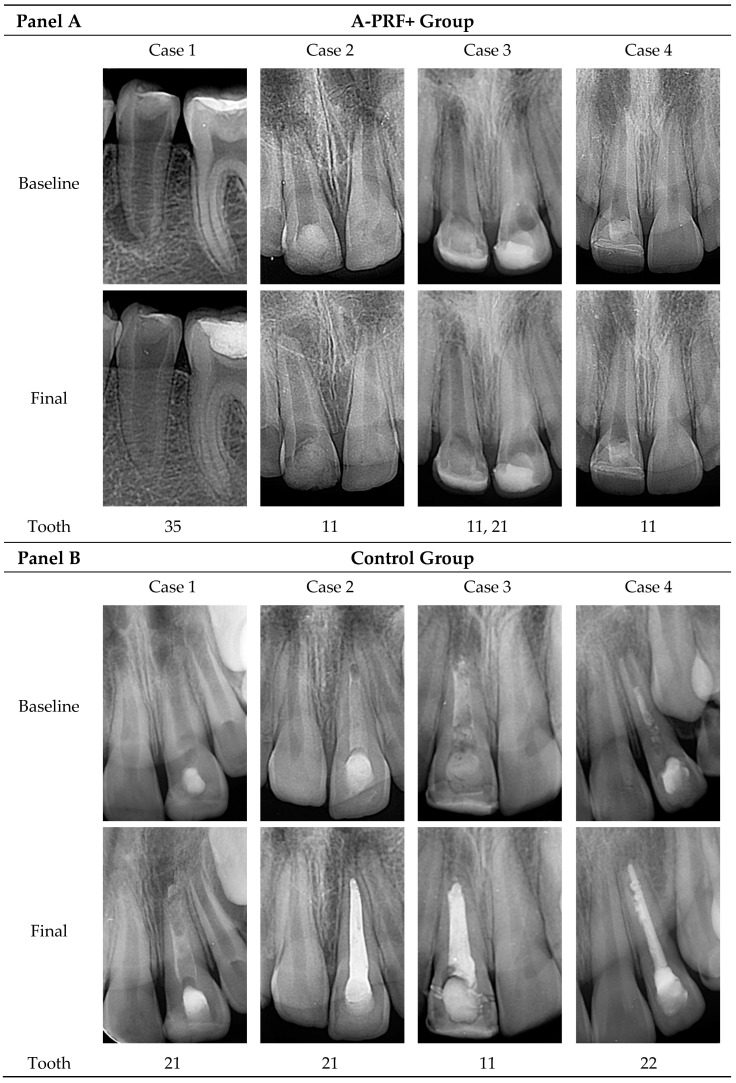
Representative periapical radiographs of immature necrotic permanent teeth treated with A-PRF+ protocol (Panel (A)) and calcium hydroxide apexification (Panel (B)). Each case shows baseline (upper row) and final (lower row) radiographs, with the designation of the treated tooth indicated below each case.
